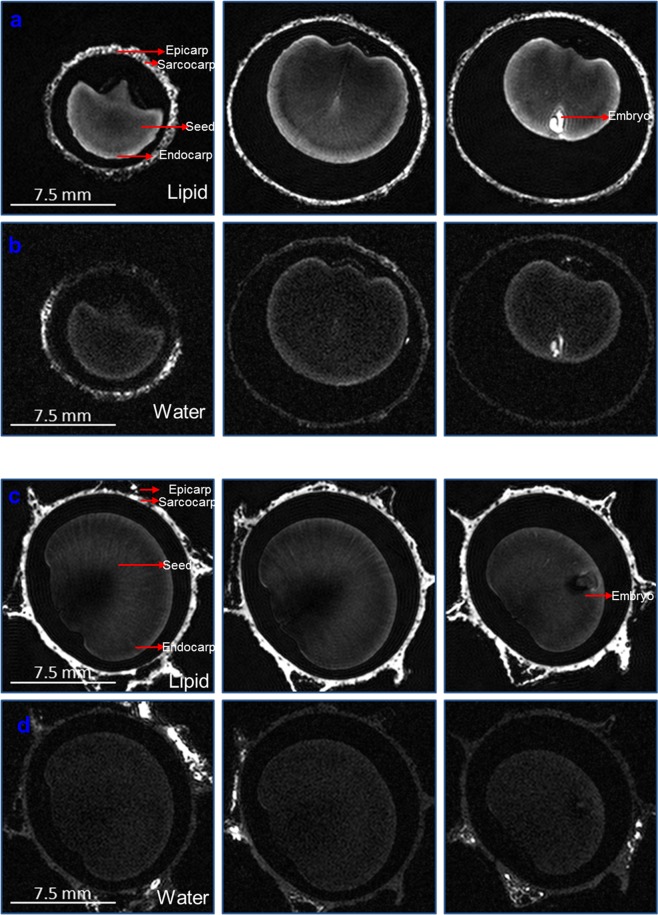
MRI images of axial slices of *S*. *repens* berries, indicating internal structures. (a,b) Represent the lipid and water distribution in wild green variety (SP), respectively and (c,d), represent the lipid and water distribution in silver variety (SL), respectively. For better visualisation of the lower intensities, a non-linear grey-scale was used which overemphasizes the lower values and chops off the highest values by setting them to the maximum value of the grey-scale.
